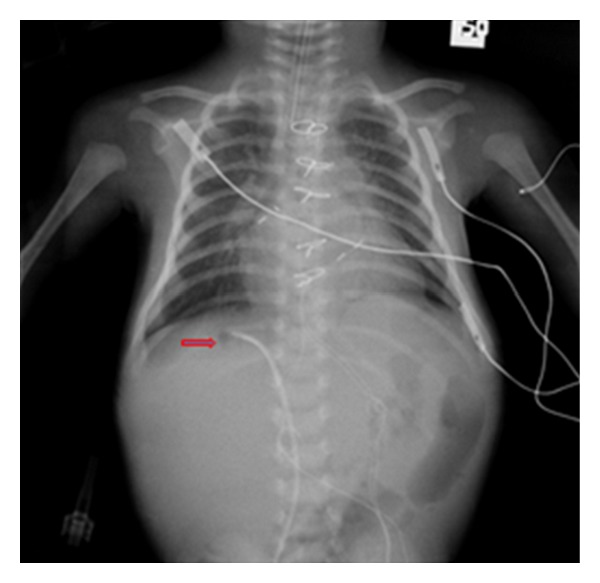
A chest radiograph on 2-month-old following cardiac surgery. A difficult venous access prompted a right femoral vein access. The tip of the catheter has entered the right hepatic vein. Note the air at the tip of the catheter, which is a potential for air embolism (arrow).
